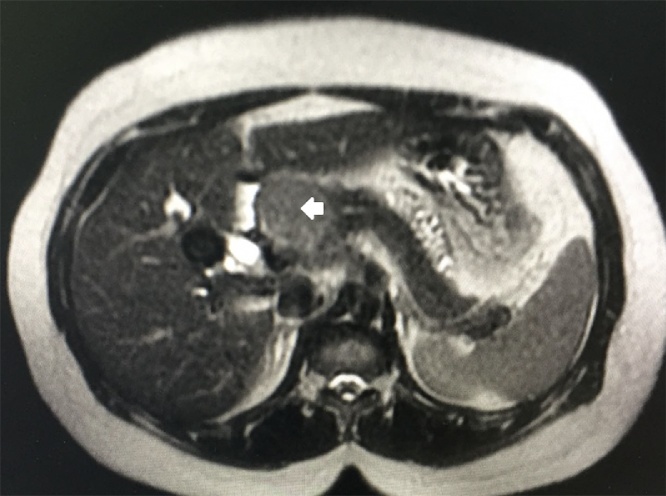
MRI axial T2. The arrow points to the mass with a signal intensity that is similar to the nomal pancreatic tissue.
